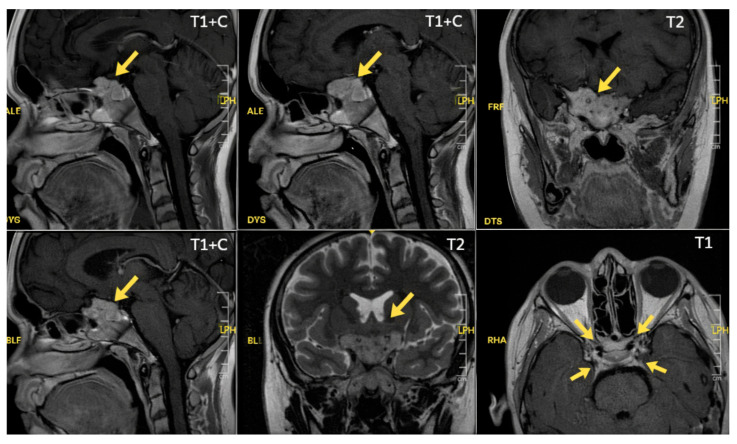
Magnetic resonance imaging (MRI) of the sellar region in a pediatric patient with Cushing syndrome shows a small nodular lesion along the left margin of the sella turcica (approximately 3 × 2 mm). The lesion appears hyperintense on T2-weighted sequences, isointense on pre-contrast T1-weighted images, and exhibits progressive enhancement following contrast administration, imaging features consistent with a pituitary microadenoma (arrows). The posterior pituitary bright spot, pituitary stalk (infundibulum), and hypothalamic structures show normal morphology and signal intensity. Source: Own work.
